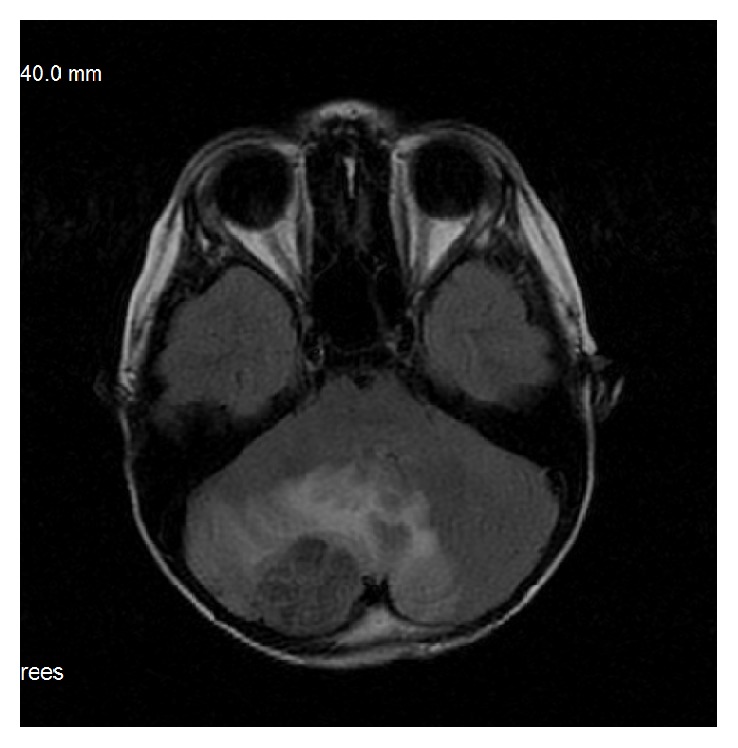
A 9-year-old boy with Ewing sarcoma of the iliac bone. One of three metastases to the brain, in infratentorial location. MRI; FLAIR.
